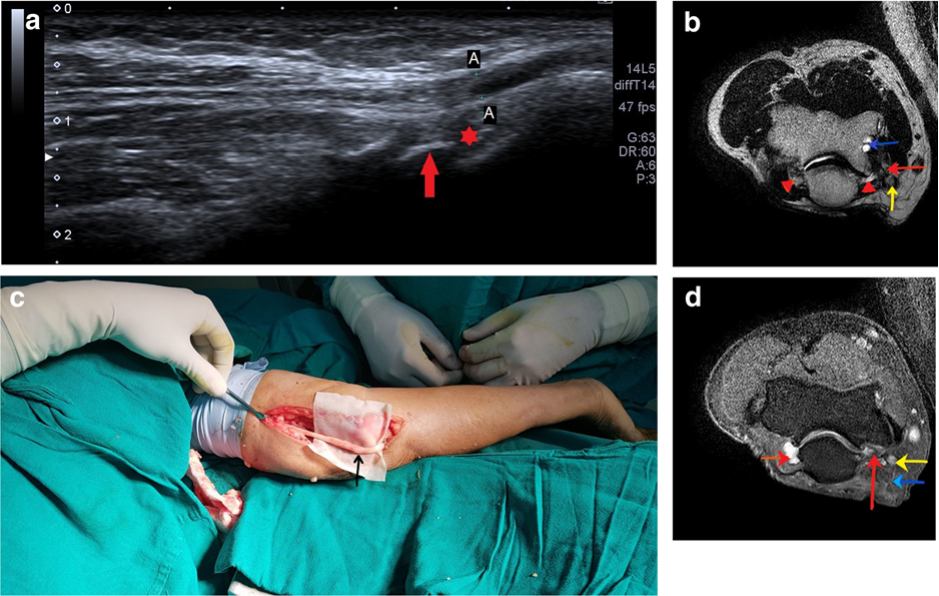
a Hypoechoic ulnar nerve (A) is seen at the level of the cubital tunnel with underlying synovial thickening (*) and cortical irregularity (red arrow). b T2-weighted MR image shows thickened hyperintense ulnar nerve (yellow arrow) with surrounding synovial thickening (red arrowhead) and joint effusion. Note is made of subchondral cysts (blue arrow) and cortical irregularity (red arrow). c A hyperintense ulnar nerve (yellow arrow), joint effusion (red arrow) and panniculitis (blue arrow) can be seen behind the median epicondyle in the cubital tunnel on this PD FS image. Chronic inflammation has led to cubital tunnel syndrome in this patient. d Intra-operative picture of the patient with cubital tunnel syndrome due to chronic inflammation shows thickened ulnar nerve
